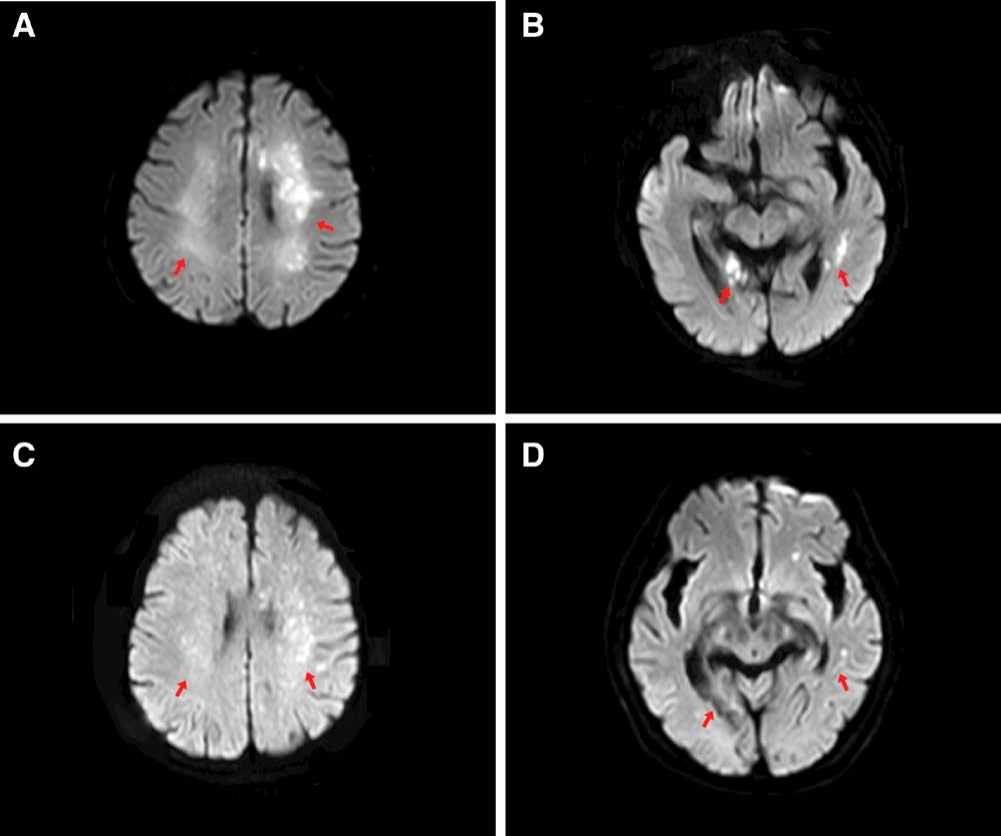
Evolution of CFE on brain MRI. (A, B) Brain MRI performed on the 1st day of neurological deterioration (day 2 post-injury). DWI sequences reveal numerous punctate hyperintense foci, some coalescing into patchy areas, distributed throughout the bilateral frontal, temporal, parietal, and occipital lobes, demonstrating the characteristic “starry sky” sign. The red arrows point to a selection of these characteristic diffuse punctate lesions. (C, D) Follow-up MRI (day 43 post-injury). DWI sequences show a significant reduction in both the number and extent of the hyperintense foci in the bilateral frontal, temporal, parietal, and occipital lobes, consistent with the patient’s clinical improvement. The red arrows point to these changed areas. CFE = cerebral fat embolism, DWI = diffusion-weighted imaging, MRI = magnetic resonance imaging.
